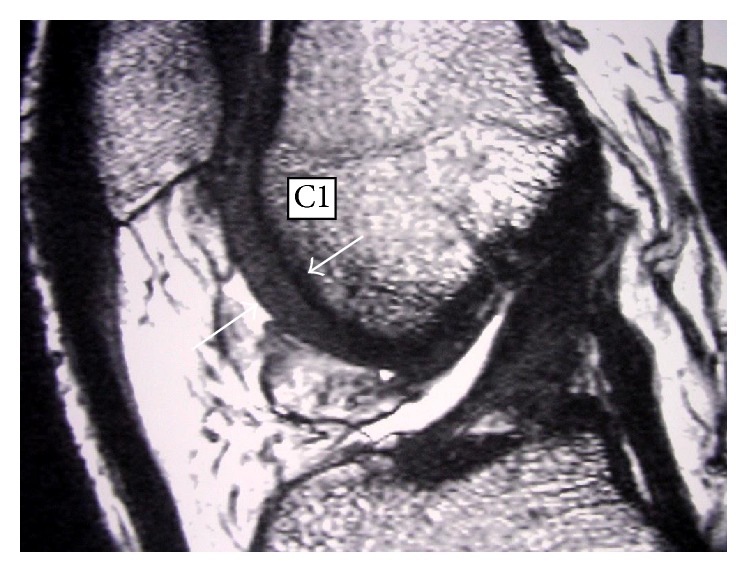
Mapping of MRI showing region that is C1. White arrows show cartilage thickness in trochlear region of lateral anterior femoral condyle.
